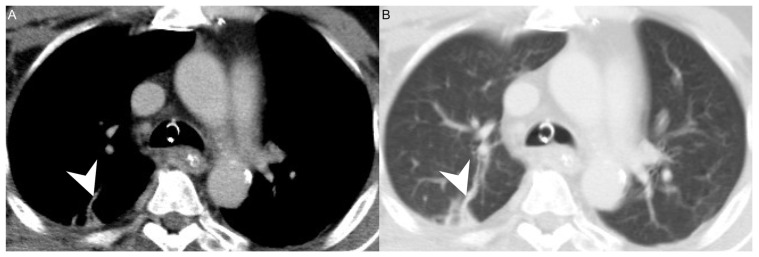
(A) CT axial view and (B) lung window of a patient with concurrent DNI and LS. Arrowhead: septic emboli.
